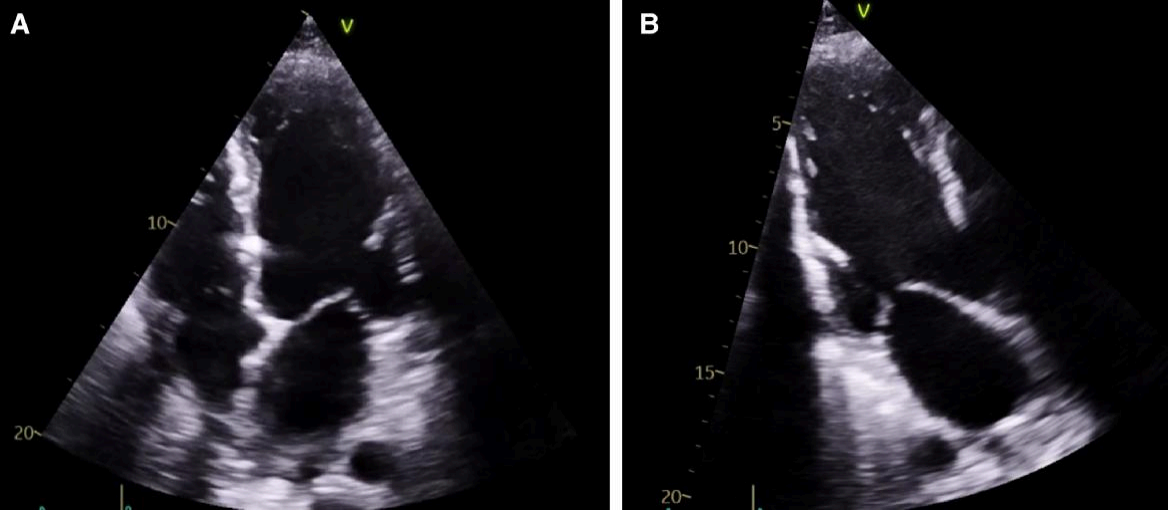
Transthoracic echocardiogram on day 77 of hospitalization. (*A*) Apical four-chamber view and (*B*) apical three-chamber view show marked hyperechogenicity in the septal and inferolateral walls, consistent with myocardial calcification. The findings correlate with impaired contractility and reduced left ventricular ejection fraction (LVEF 33%).
